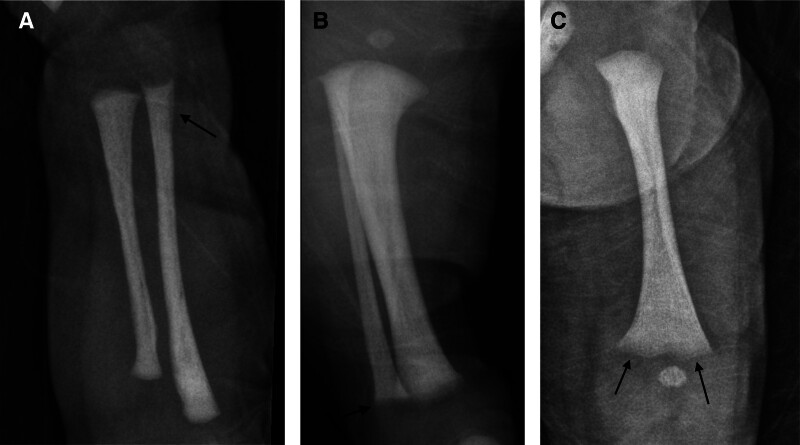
A: Radiograph of the right forearm demonstrates cupping of the distal radial metaphysis as well as periostitis (black arrow). B: Radiograph of the right tibia and fibula demonstrates irregularity of the distal tibial and fibular metaphyses (black arrow). C: Radiograph of the left femur demonstrates loss of mineralization in the distal femoral metaphysis as well as loss of the zone of provisional calcification in the subchondral bone (black arrows).
